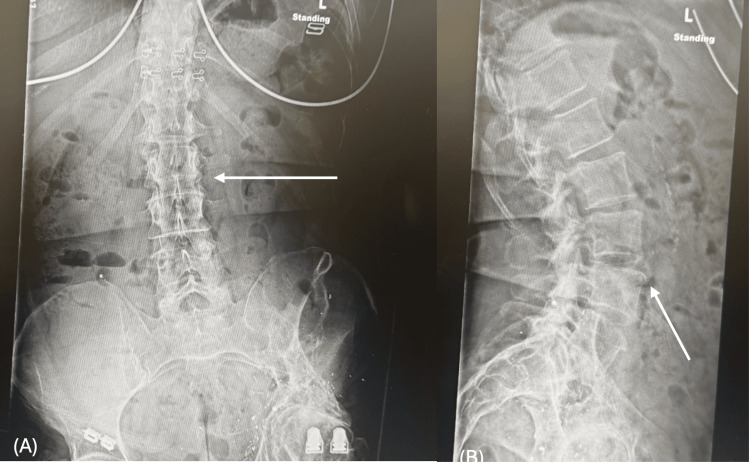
Preoperative lumbar spine radiographs. (A) Anteroposterior view of the lumbar spine showing a gentle compensatory curvature related to pelvic obliquity.
